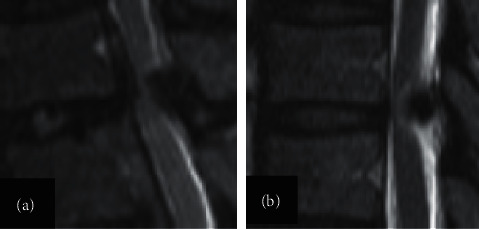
The shape on the sagittal MRI of TOLF. Sagittal T2-weighted MR images demonstrating morphological classifications of TOLF: (a) beak type and (b) round type.
